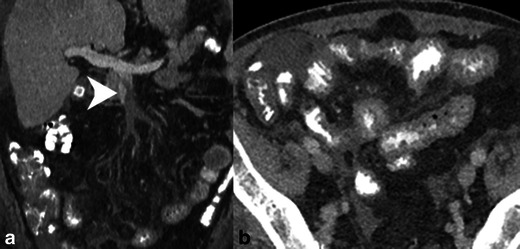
SMV thrombosis. a Contrast-enhanced coronal reformatted CT demonstrates occlusive thrombus in the SMV near the portal confluence (*white arrowhead*). b Axial CT image demonstrates diffuse small bowel wall thickening secondary to venous congestion and ischaemia
